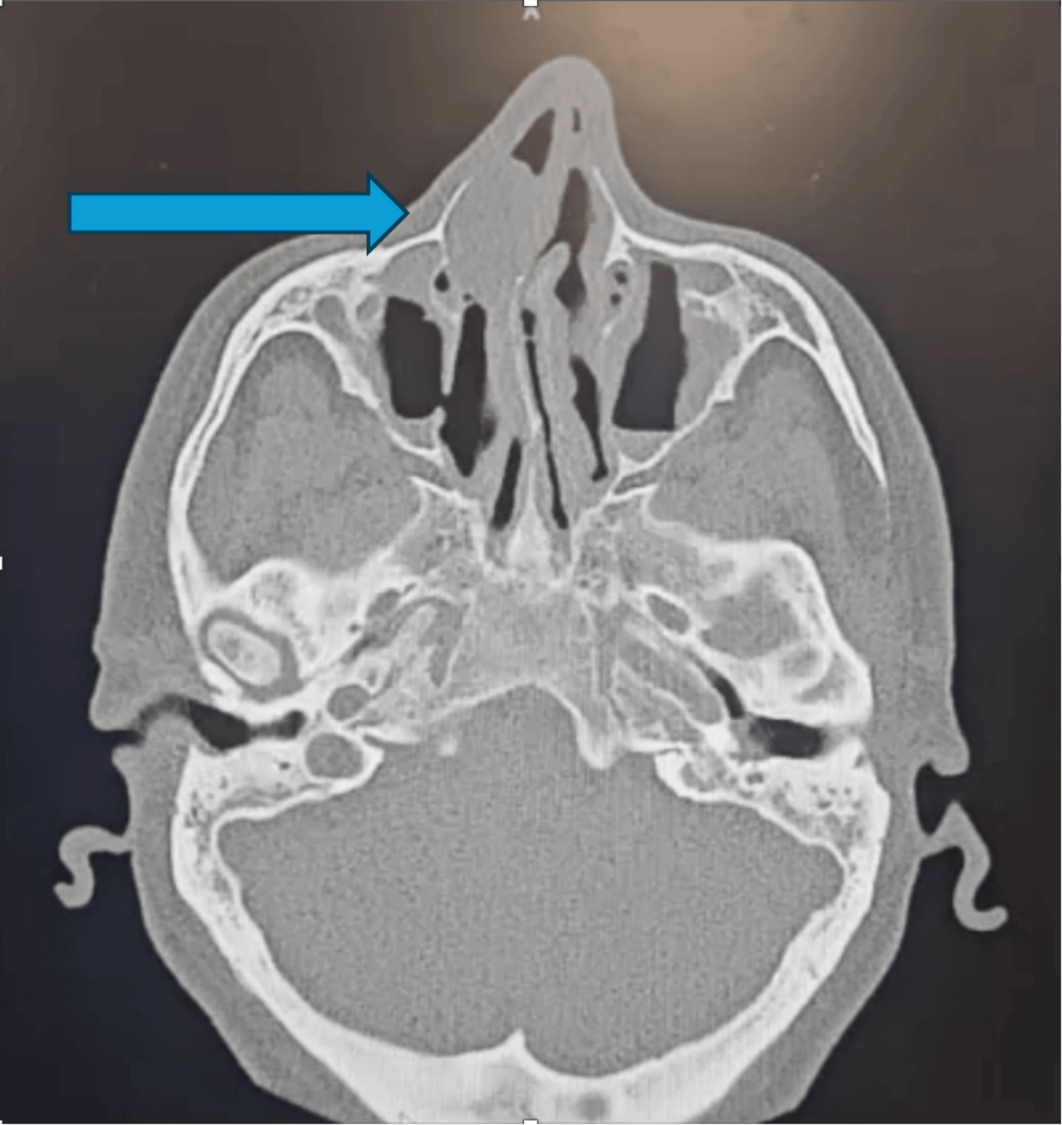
CT sinuses: dominant right-sided nasal cavity lesion causing obstruction on a background ofbilateral diffuse mucosal thickening with polypoid changes
